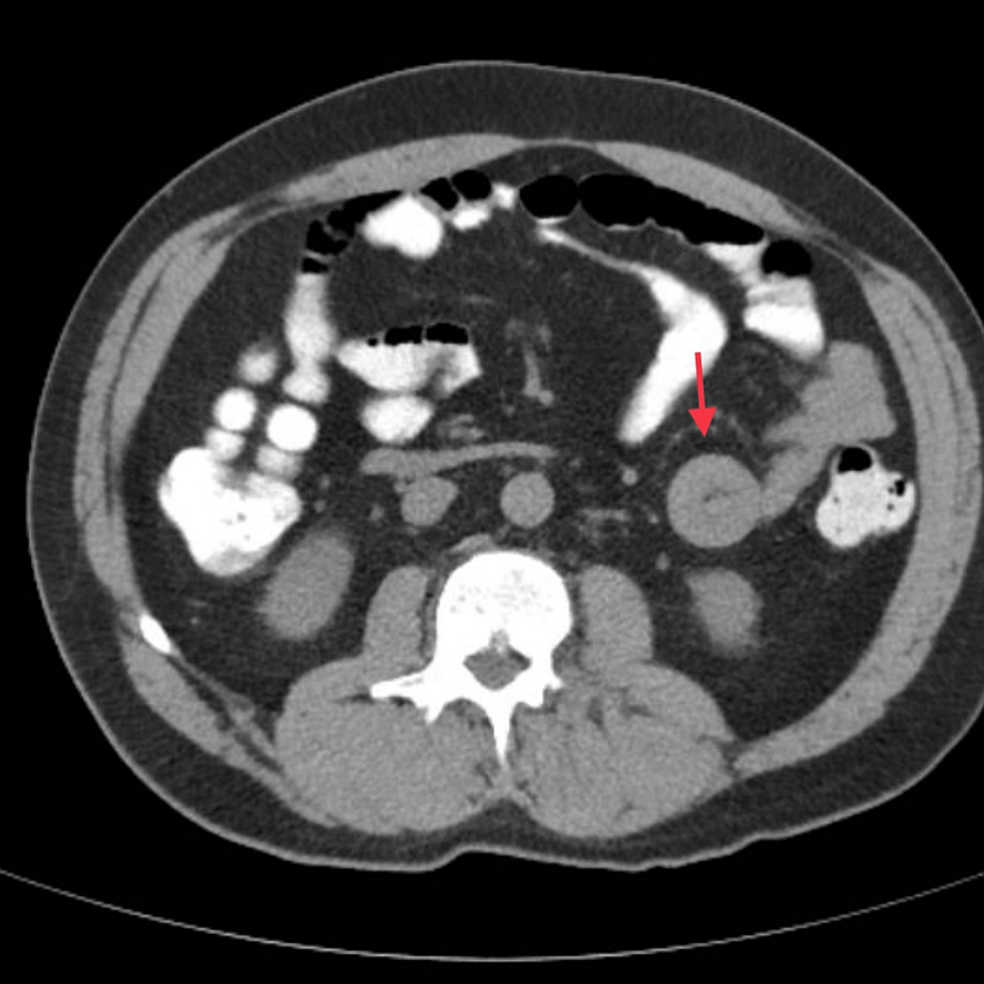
CT scan demonstrating the classical “target” sign morphology of intussusception.
